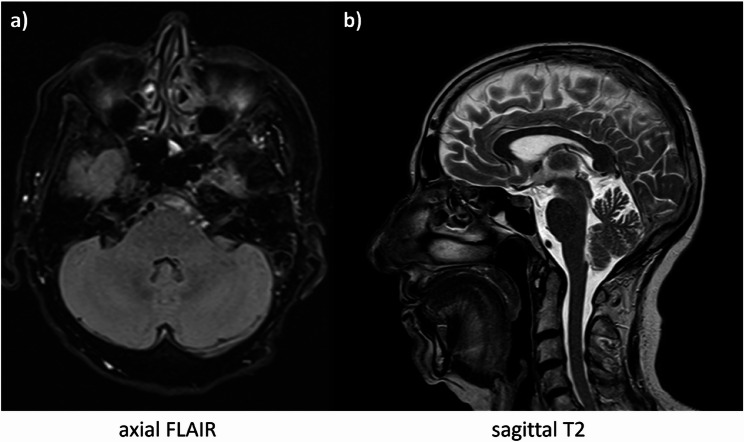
MRI of the patient’s neurocranium. Axial FLAIR- (a) and sagittal T2 (b) sequences of thin-layered MRI with normal presentation of infratentorial brain structures. Notably, uni- or bilateral hyperintense lesions (usually a sign of vasogenic edema), or signs of cerebellar atrophy were absent despite significant symptoms for more than two weeks. FLAIR = fluid-attenuated inversion recovery.
